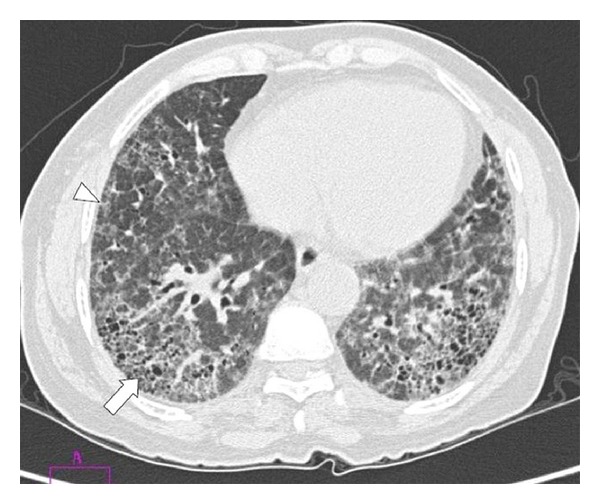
HRCT demonstrating interstitial thickening (arrow head) and honeycombing (arrow) in basal regions of lung.
